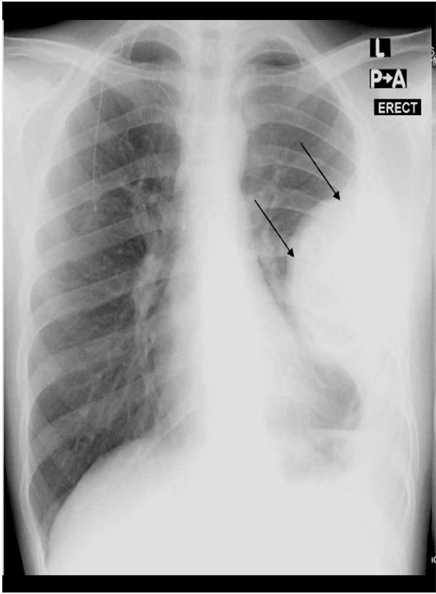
Chest radiograph showing a large pleural-based mass in the left hemithorax (arrows), with underlying rib destruction and a pleural effusion.
